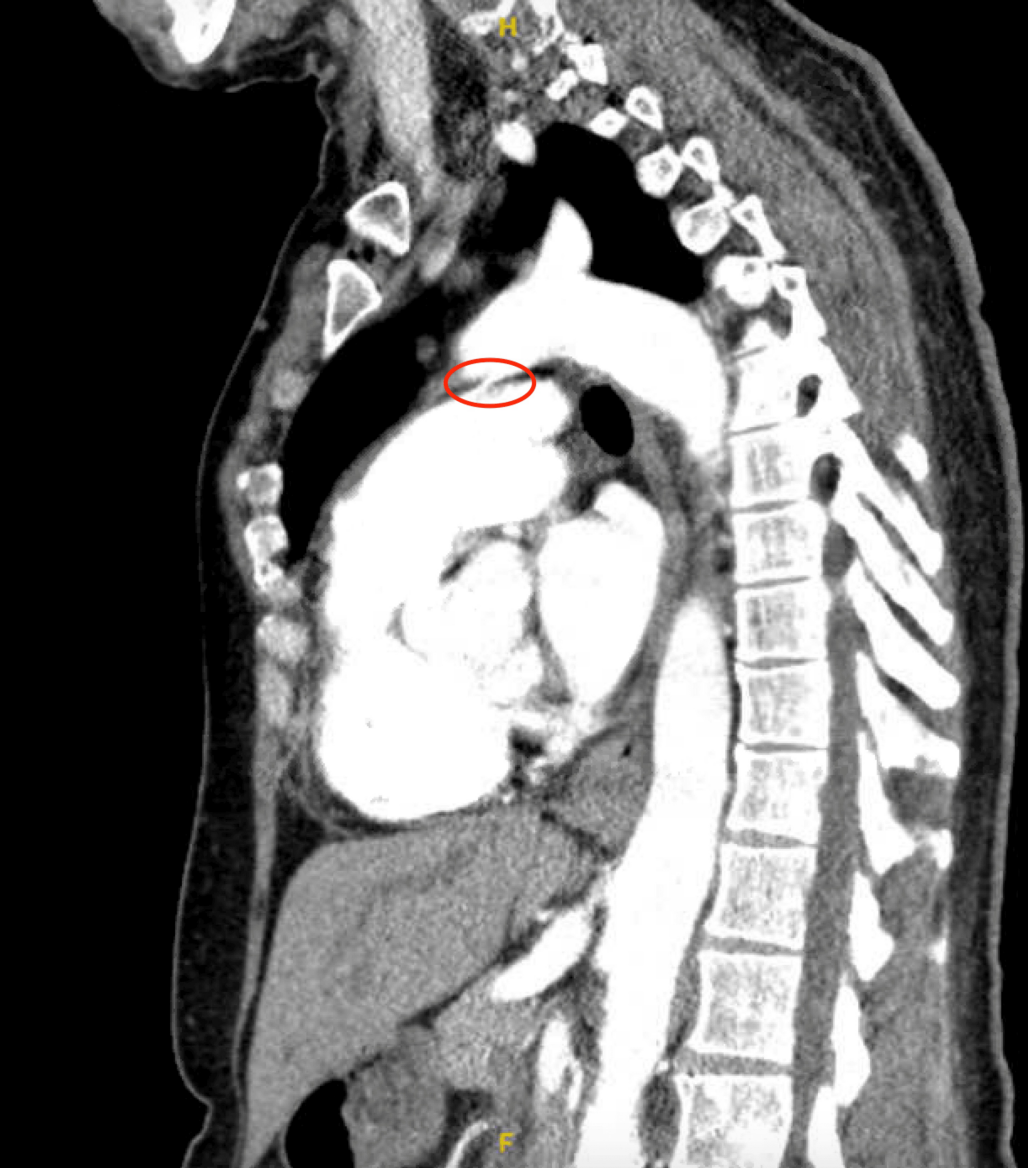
Contrast-enhanced CTA of the thoracic aorta (sagittal view) demonstrating a small PDA (red circle) in the early postpartum period The PDA is seen arising distal to the left subclavian artery and connecting to the proximal pulmonary artery. The ascending aorta, aortic arch, and descending thoracic aorta are visualized without evidence of dissection. This study was obtained during evaluation of postpartum chest pain and newly reduced left ventricular ejection fraction. CTA: computed tomography angiography; PDA: patent ductus arteriosus
